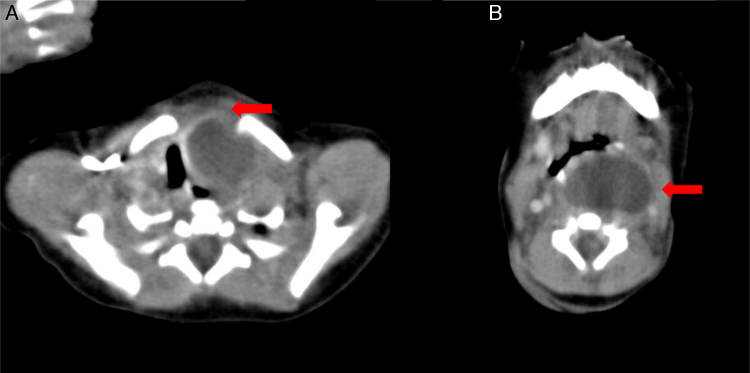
Preoperative computed tomography scan shows 4.5 cm × 3 cm size unilocular low density cystic mass which is extended into superior mediastinum with compressing the trachea. A, red arrow: intrathoracic lesion. B, red arrow: superior lesion.
